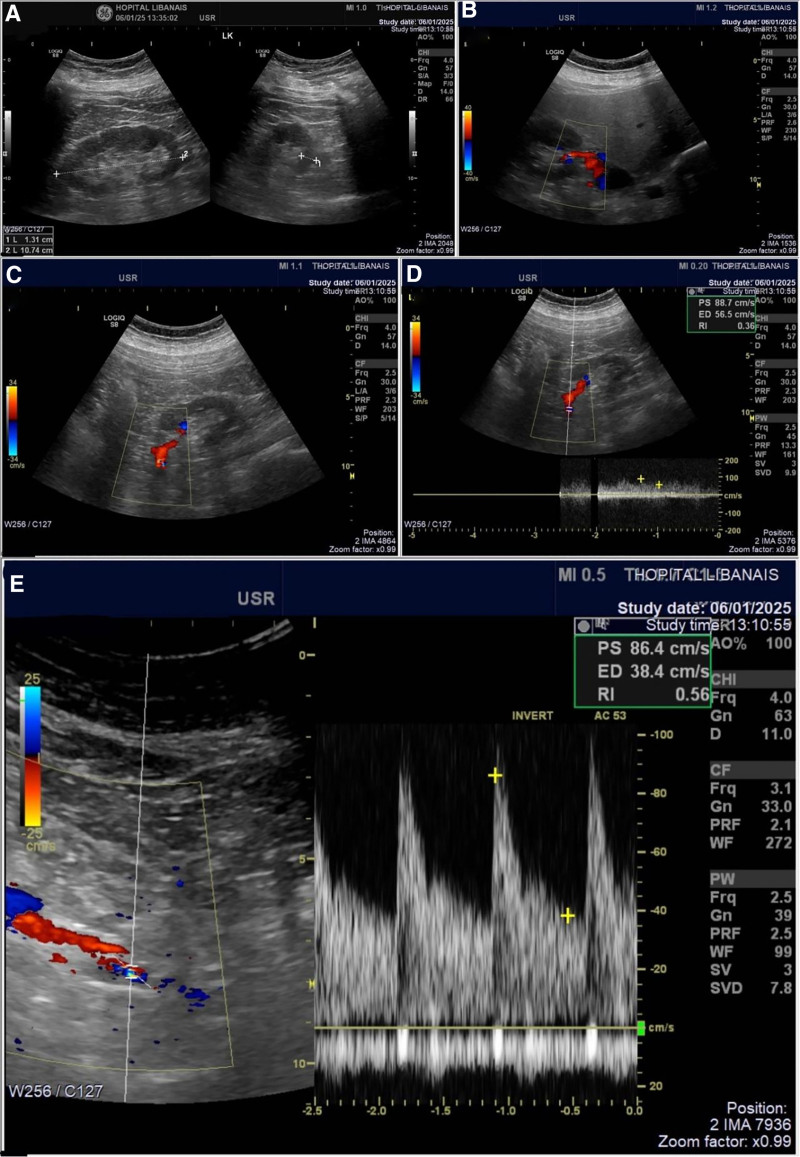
(A–E) Renal Doppler ultrasound showing normal patency of the renal arteries and veins bilaterally with normal global vascularization.
